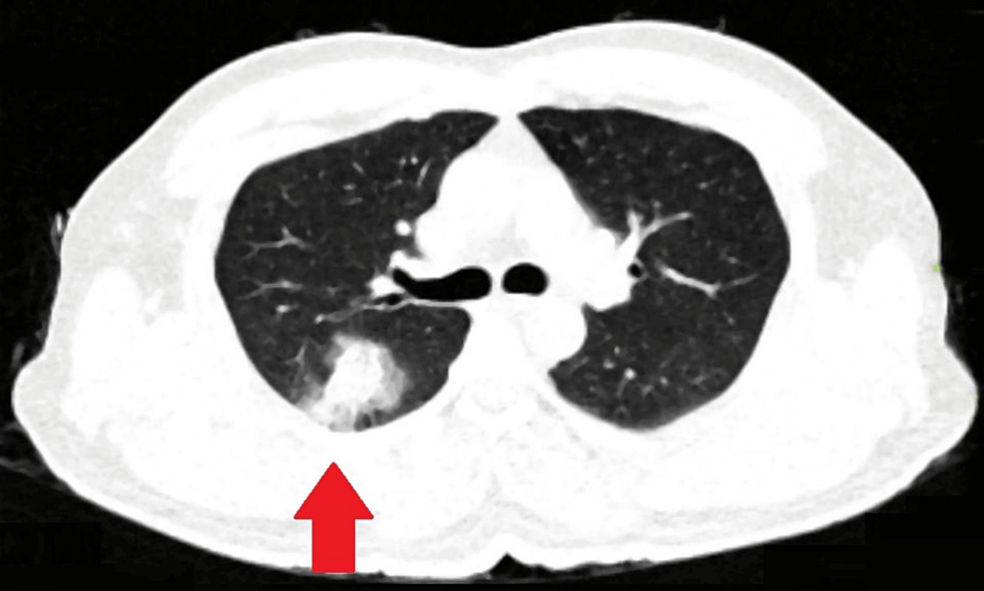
High-resolution computed tomography showing a well-defined consolidation measuring 2.6 × 2.2 × 2.4 cm with surrounding ground glass opacity in the superobasal segment of the lower lobe on the right side (the classical halo sign)
